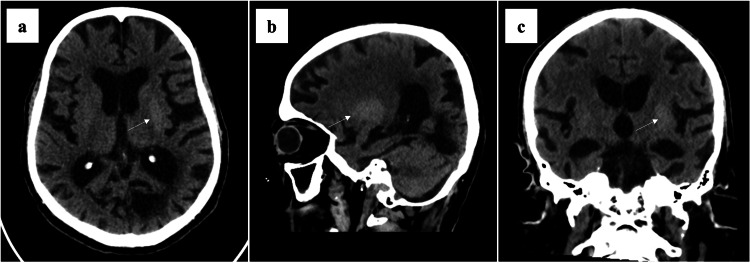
Axial (a), sagittal (b), and coronal (c) non-contrast brain CT images demonstrating subtle hyperdensity in the left putamen, contralateral to the patient’s right-sided hemichorea-hemiballismus, as highlighted by arrows CT: Computed tomography
